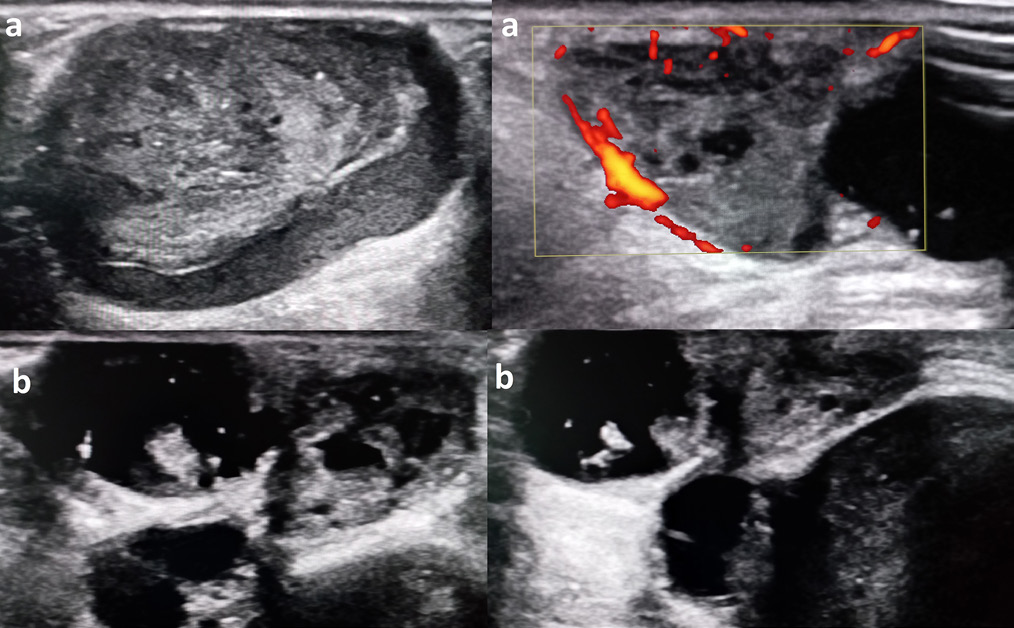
Ultrasound images showing a multilobulated oval-shaped mass of the cutaneous and subcutaneous tissue, with heterogeneous solid components (a) and cystic components, some of these cysts contain internal echoes (b).
